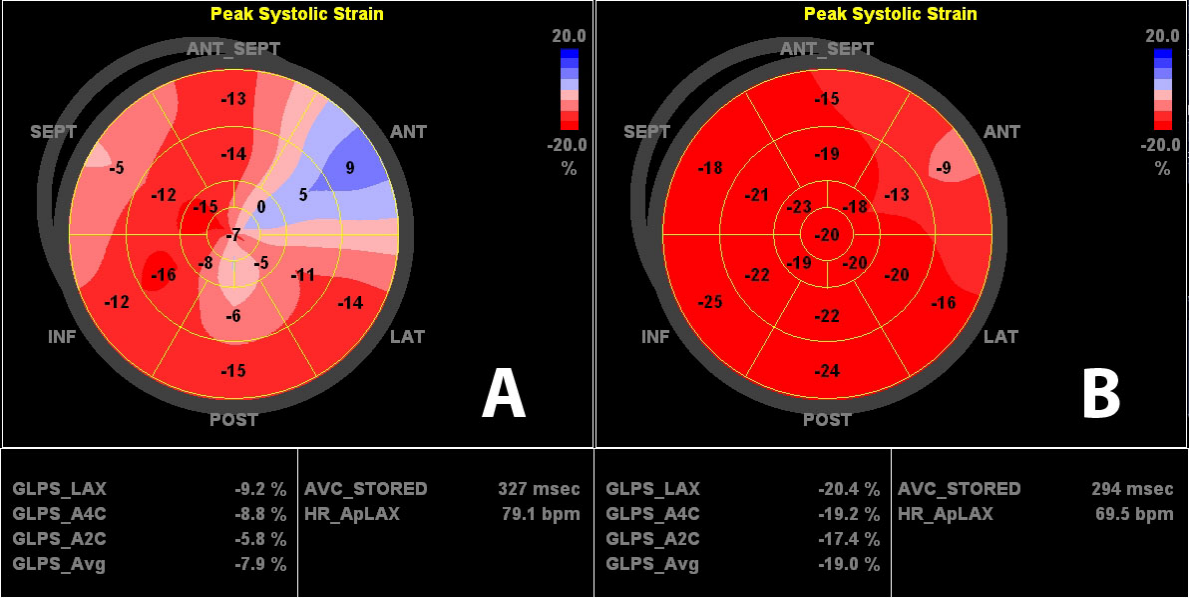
Apical four chamber view longitudinal strain curves before (top) and one week post TAVI (bottom). There is an increase in longitudinal strain in all segments.
